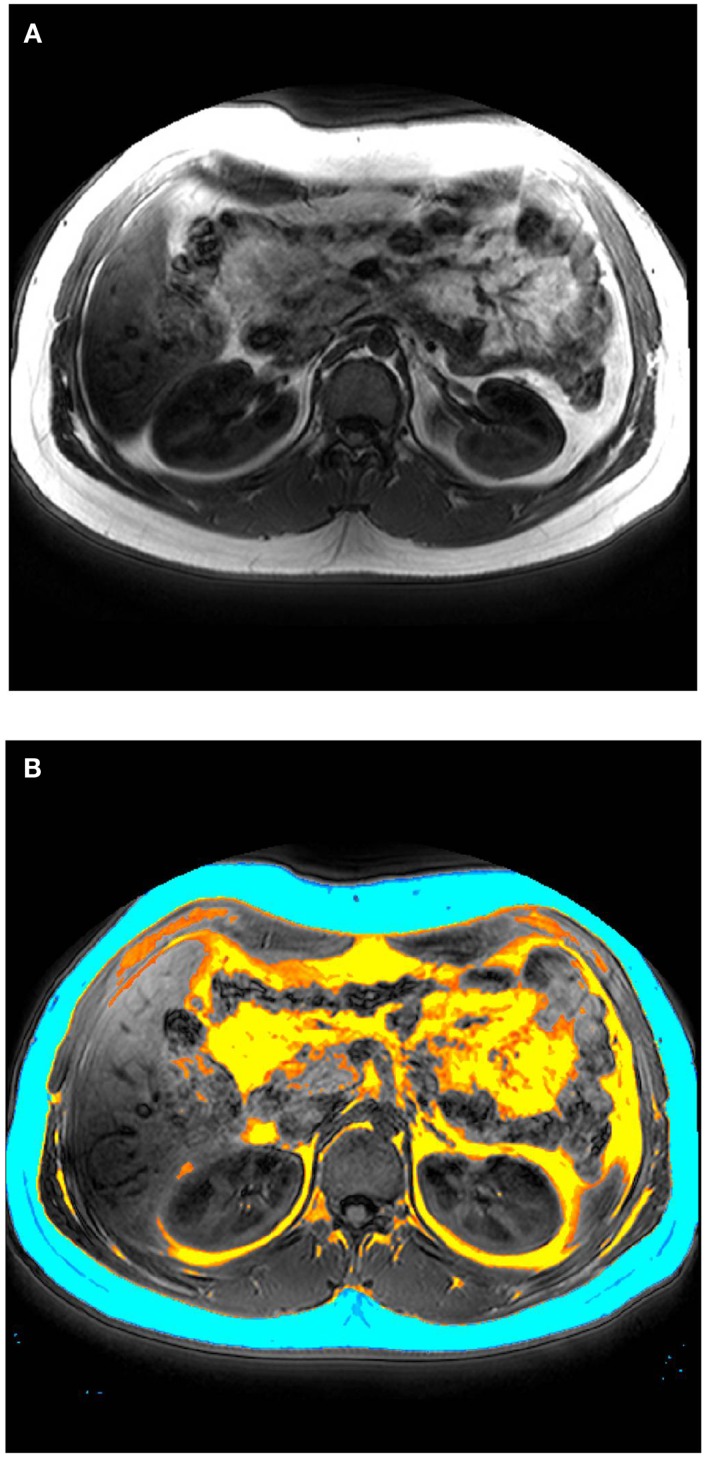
(A) Representative MRI image (axial plane) performed on a 3-T clinical scanner. (B) Total visceral fat (yellow), subcutaneous fat (blue), and intramuscular fat (orange) manually segmented.
